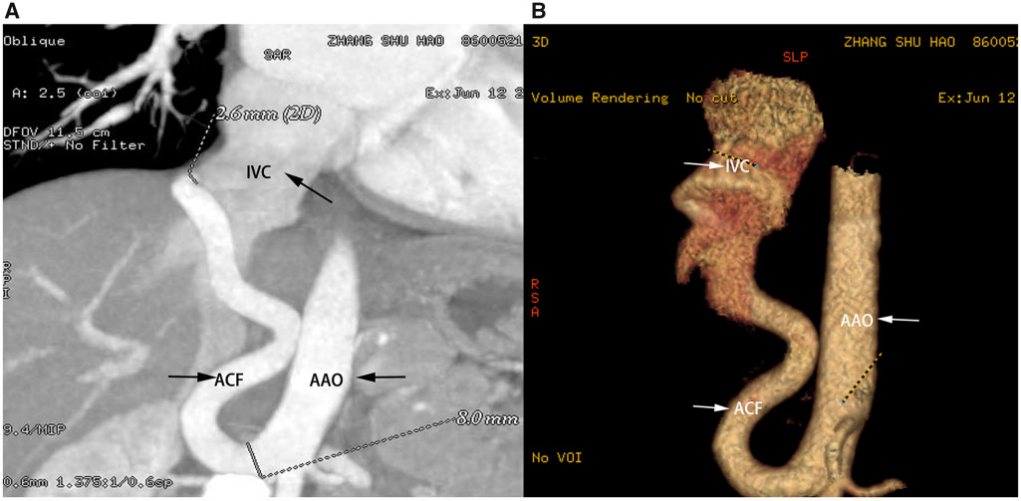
Computed tomography angiography showing a tortuous, large, and very long fistula between the abdominal aorta and inferior vena cava. IVC: Inferior vena cava; AAO: Abdominal aorta; ACF: Aortocaval fistula.
